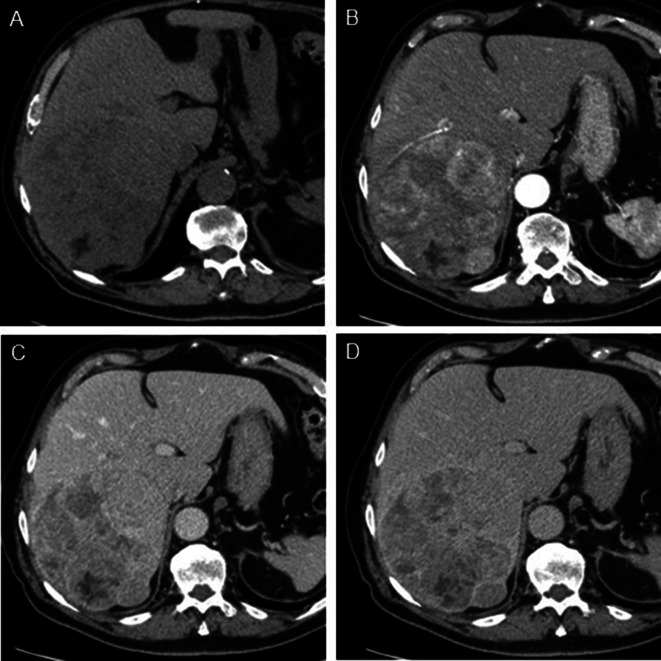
HCC on multiphasic contrast enhanced CT. Axial non-contrast (A), arterial phase (B), portal venous phase (C), and delayed phase (D) post contrast CT images show a large heterogeneous right hepatic lobe mass with arterial phase hyperenhancement, washout, and enhancing capsule diagnostic of HCC
